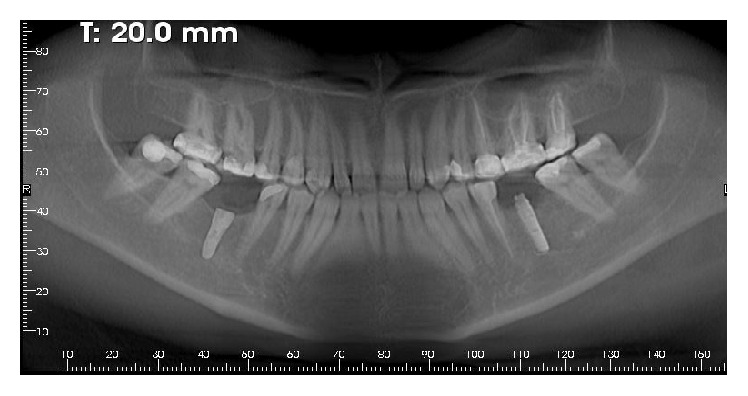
Panoramic X-ray after implant placement.
